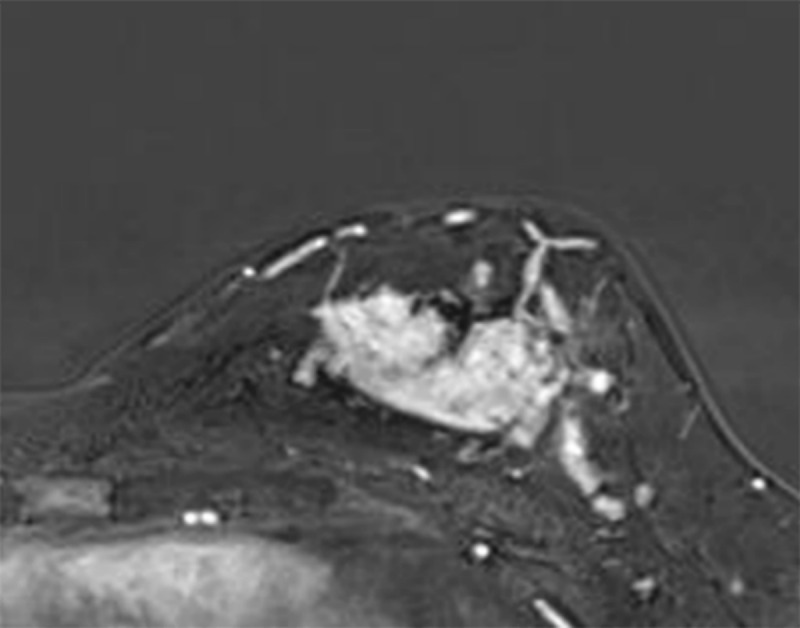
A large mass involving the left breast on DCE-MRI.
